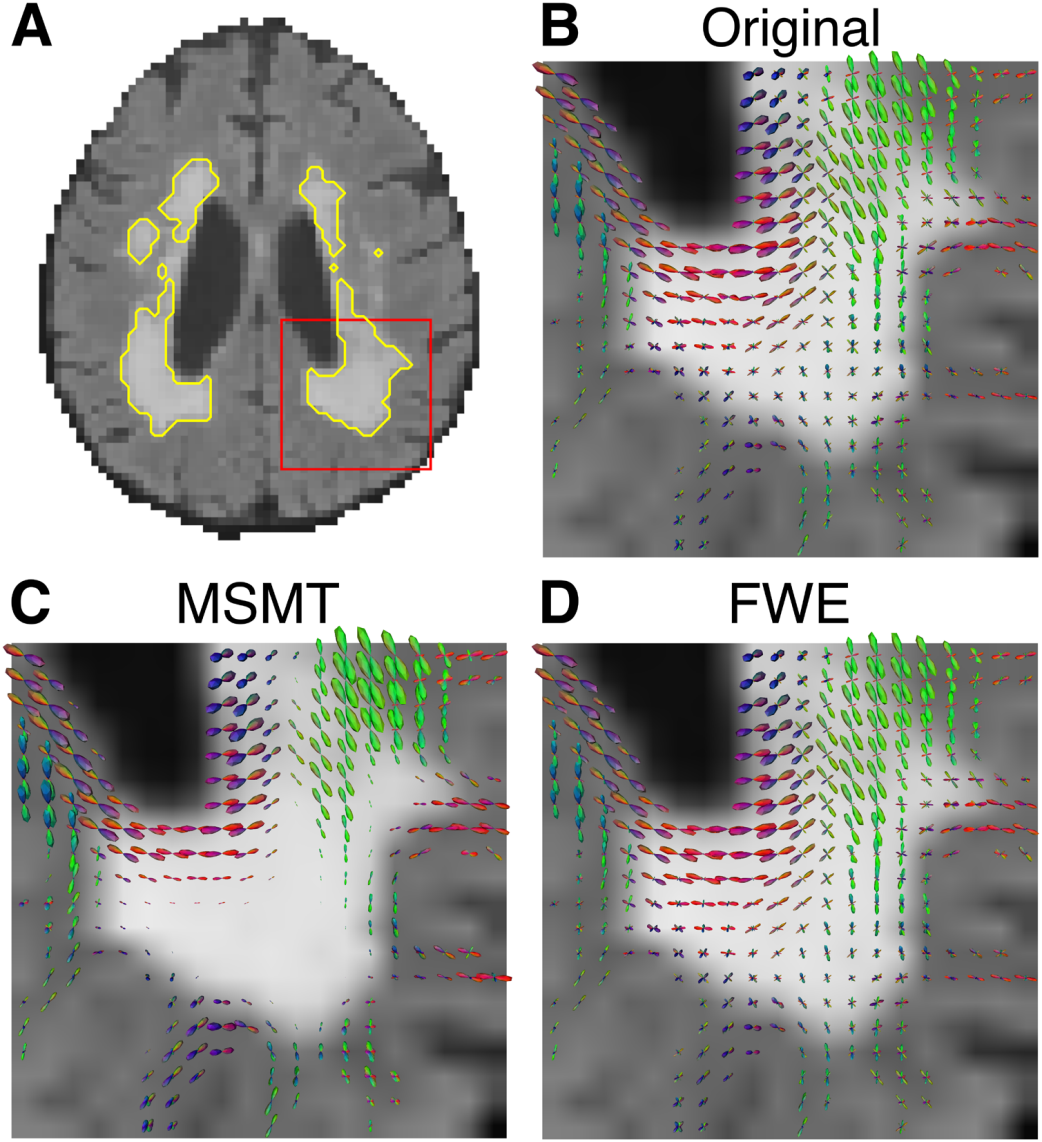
Fiber orientation distribution functions (fODFs) in white matter hyperintensities (WMH) for an example participant. (A) Axial FLAIR image with WMH regions outlined in yellow. The red box indicates the region enlarged in panels (B–D). (B) fODFs derived from the Original diffusion data, (C) fODFs after MSMT processing, and (D) fODFs after FWE processing are shown for the zoomed-in region.
